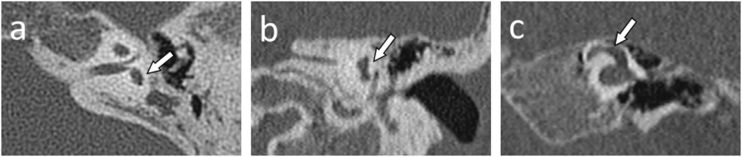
Semicircular canals and vestibule malformations identified in computed tomography evaluation and presented in dedicated planes. Panel a and b) represent the same patient (case #2) with a hypoplastic vestibule and the absence of all three semicircular canals. Panel c (case #10) shows a dysmorphic superior semicircular canal with an accompanying dilated vestibule.
